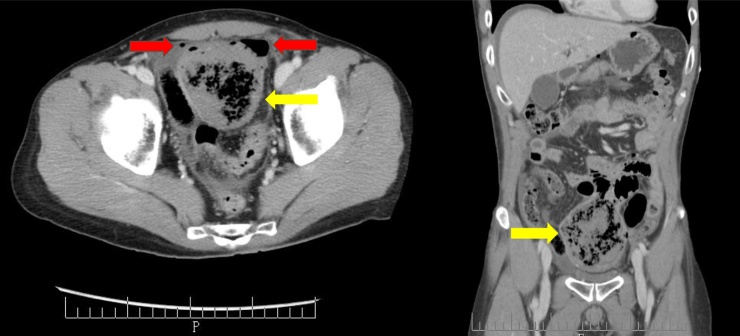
Computed tomography findings. A 12-cm cystic dilatation is observed in the small intestine, and pooled residues are seen inside the abdominal cavity (yellow arrows). Free air is found in the abdominal cavity (red arrows).
